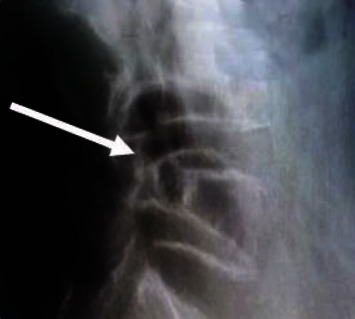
Probe position under ultrasound guidance.
